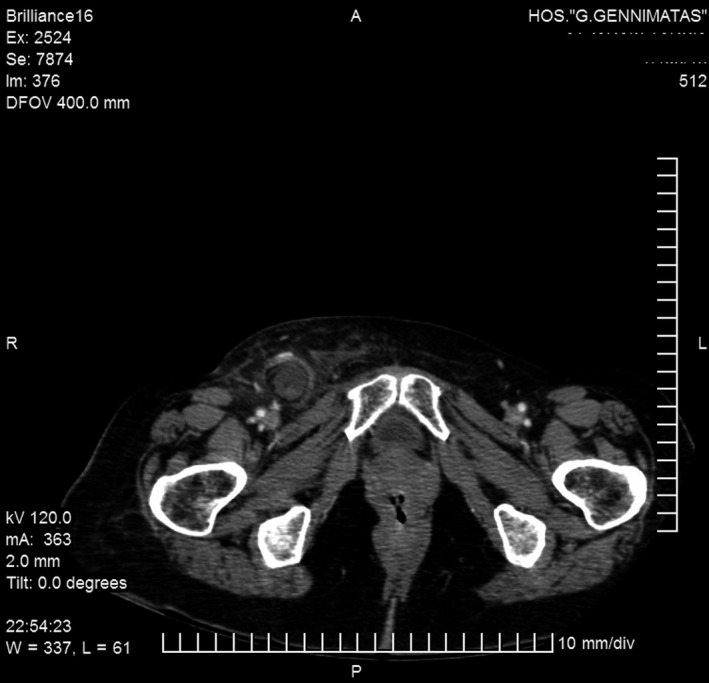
Preoperative CT scan of the abdomen‐pelvis showing a right femoral hernia containing the swollen appendix and fluid collection in the femoral canal
